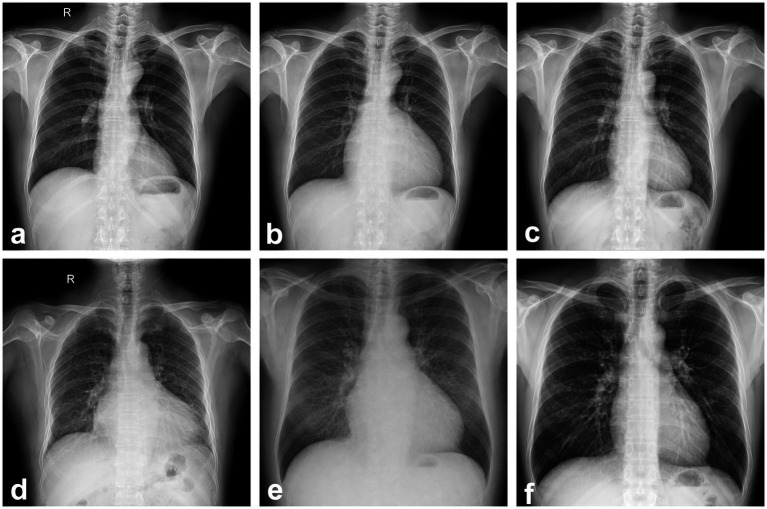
Representative cardiomegaly case and matched normal conditioning radiograph. (a) Normal conditioning radiograph from a 55-year-old man used for image-conditioned generation. (b,c) Image-conditioned synthetic radiographs generated by the GPT-image and Gemini-image models, respectively. (d) Real disease-positive radiograph from a 53-year-old man with cardiomegaly, with a cardiothoracic ratio of 0.65. (e,f) Text-only synthetic radiographs generated by the GPT-image and Gemini-image models, respectively.
